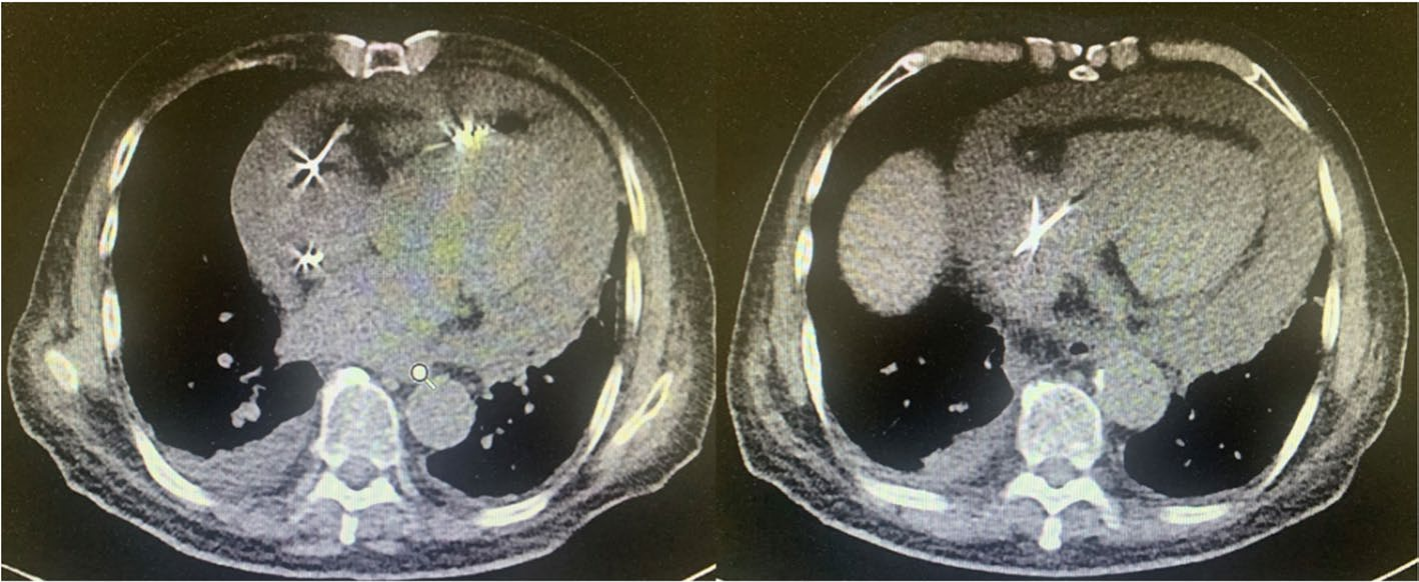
Chest CT: Large pericardium effusion, bilateral pleural effusion, partial expansion of both lungs, rule out perforation
